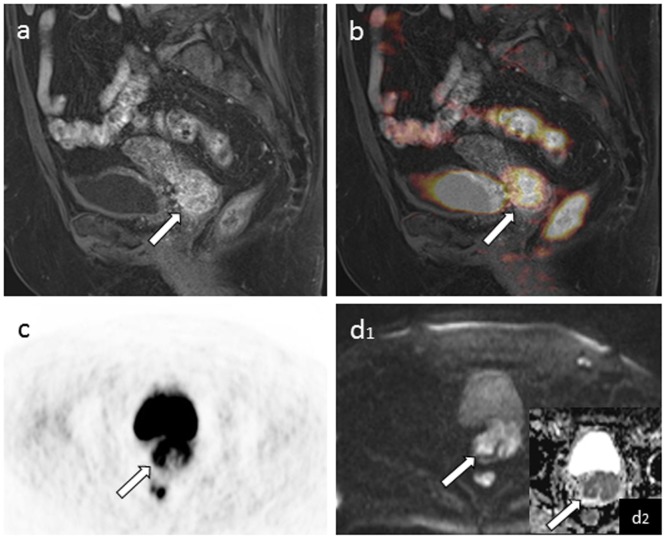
T1 weighted post-contrast sagittal 3D VIBE (a) and fused PET/MRI image (b) of an inhomogeneously contrast-enhancing and FDG avid cervical cancer lesion. Image c and d show corresponding tracer uptake in PET (c) and restricted diffusion in DWI (d_1_) with low signal intensity on the corresponding ADC map (d_2_).
